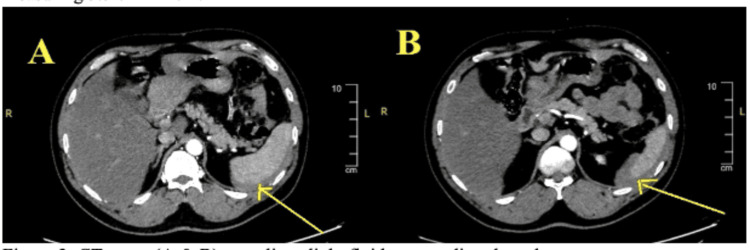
CT scans revealing intraperitoneal fluid surrounding the spleen (A and B).
